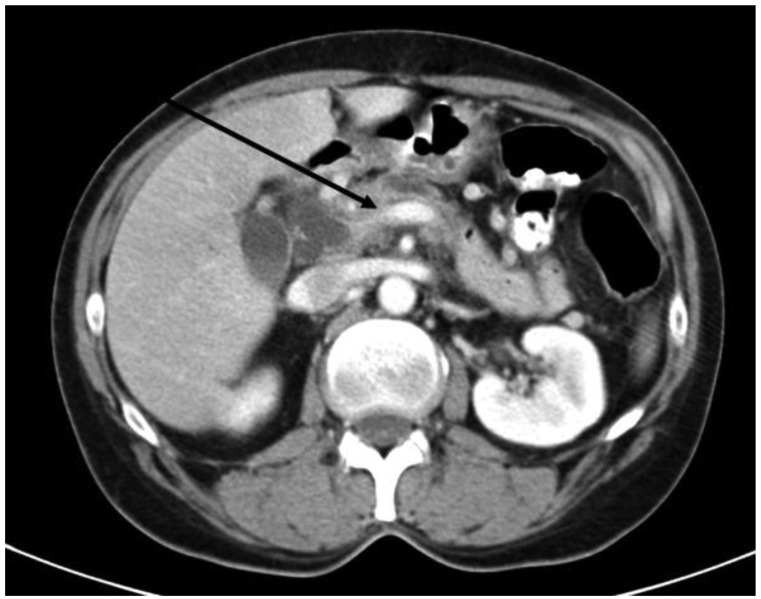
CT scan showing pancreatic head cancer with infiltration of the portal vein confluens (black arrow). Technically resectable finding (partial duodeno-pancreatectomy with segmental portal vein resection).
